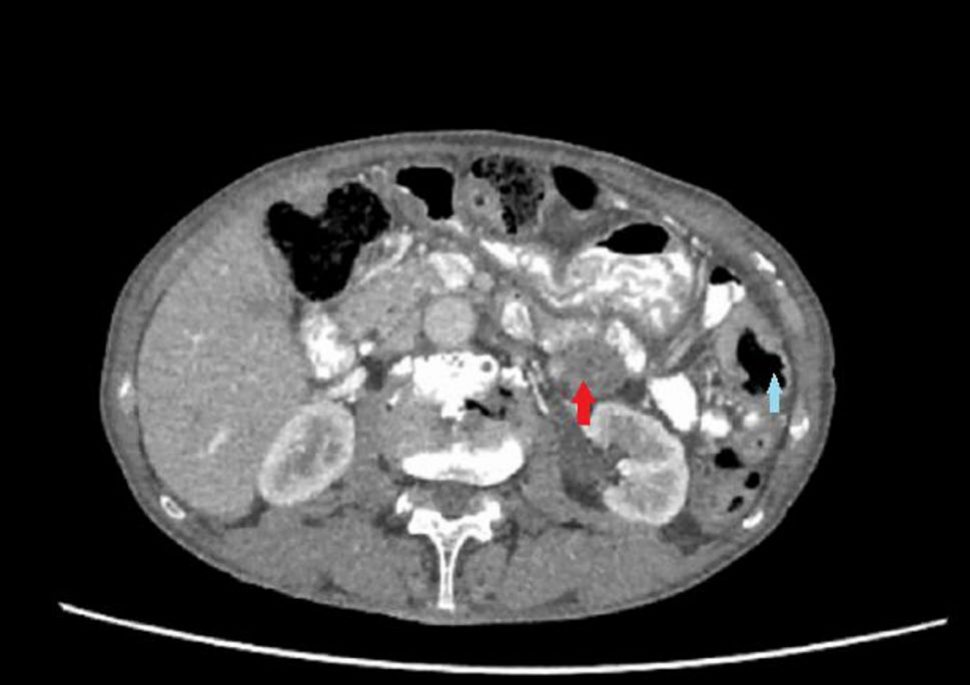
Computed tomographic scan shows an enlarged lymph node in the central mesentery (red arrow) with a splenic flexure tumor (blue arrow)
